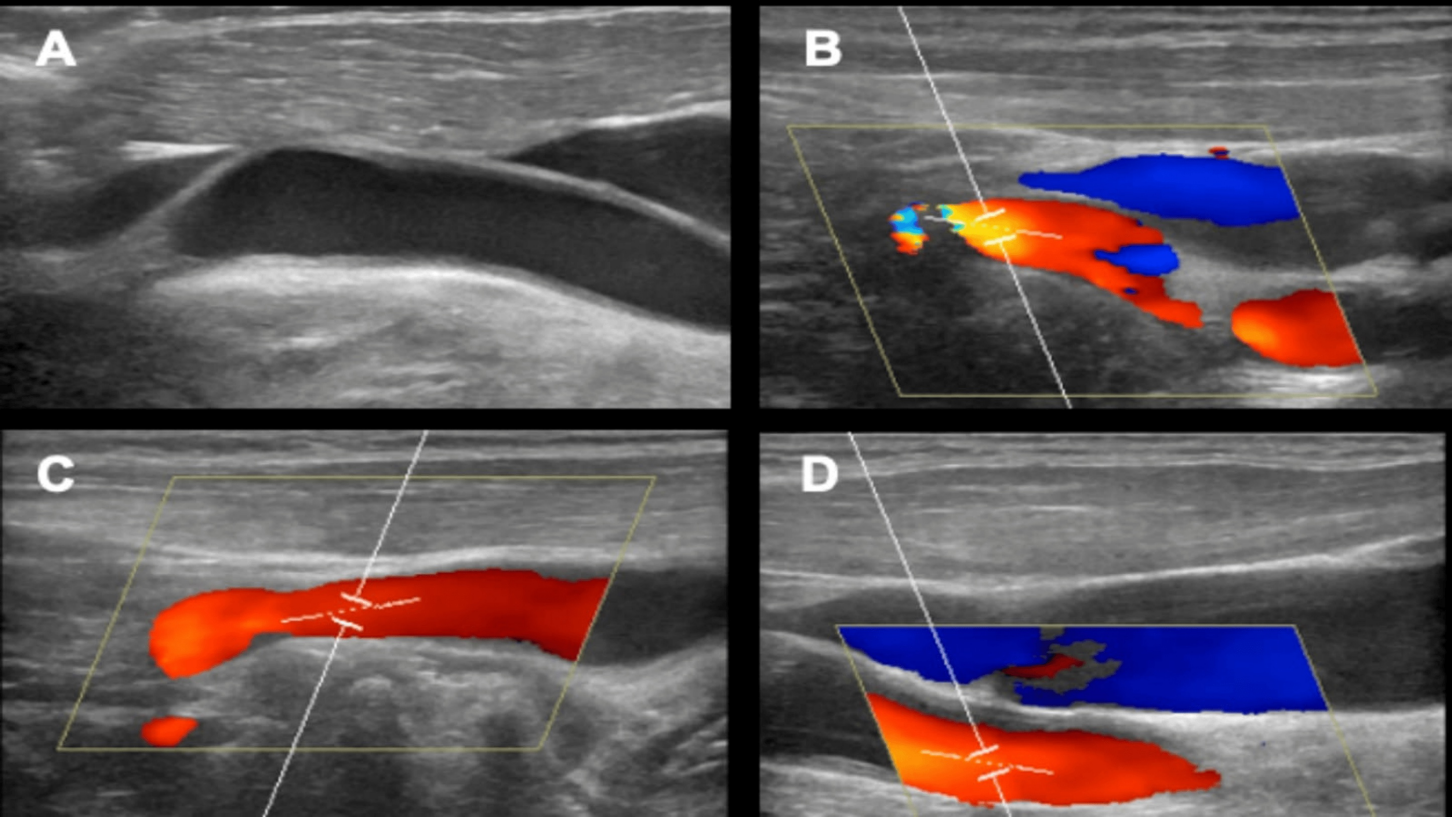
Carotid artery and vertebral ultrasound image of the patient Morphological study: no anatomical changes relevant (A: carotid morphological study). Doppler study: no stenosis with hemodynamic significance. The vertebrae are permeable and have a cephalic flow direction (B: left internal carotid artery; C: right common carotid artery; D: left common carotid artery)
